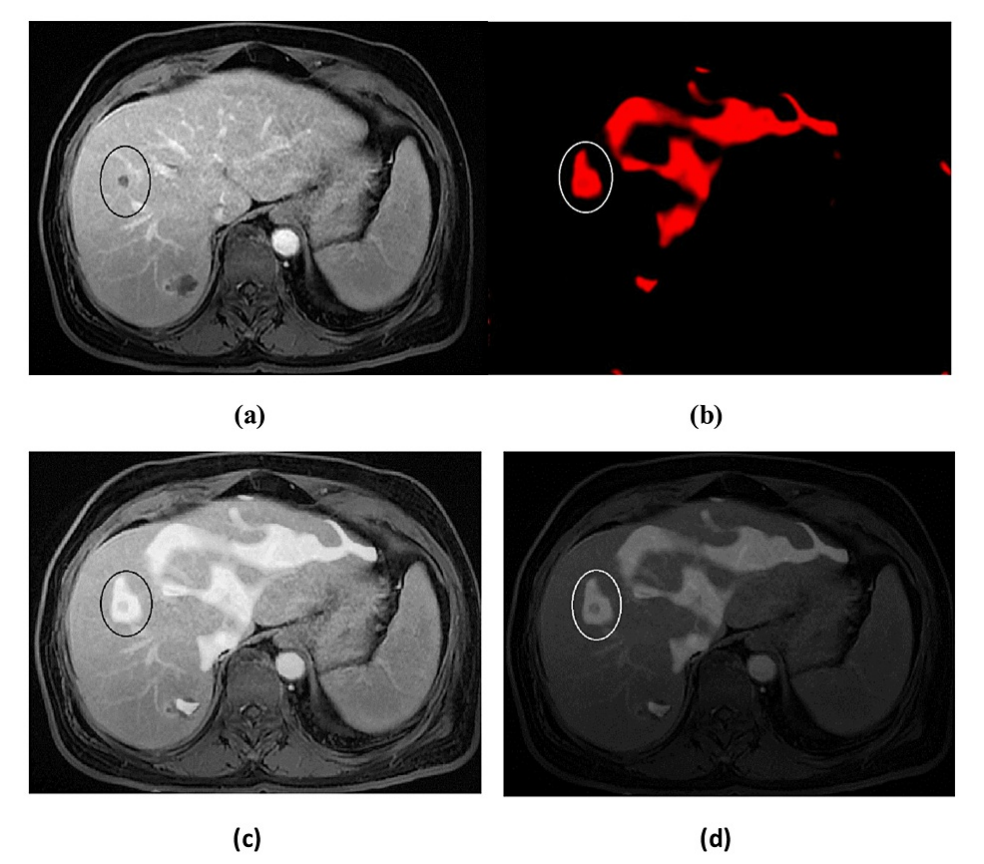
MRI-MRE fusion. (a) Liver MRI. (b) Liver MRE. (c) Bright fused image. (d) Dark fused image. MRI = magnetic resonance imaging; MRE = magnetic resonance elastography
